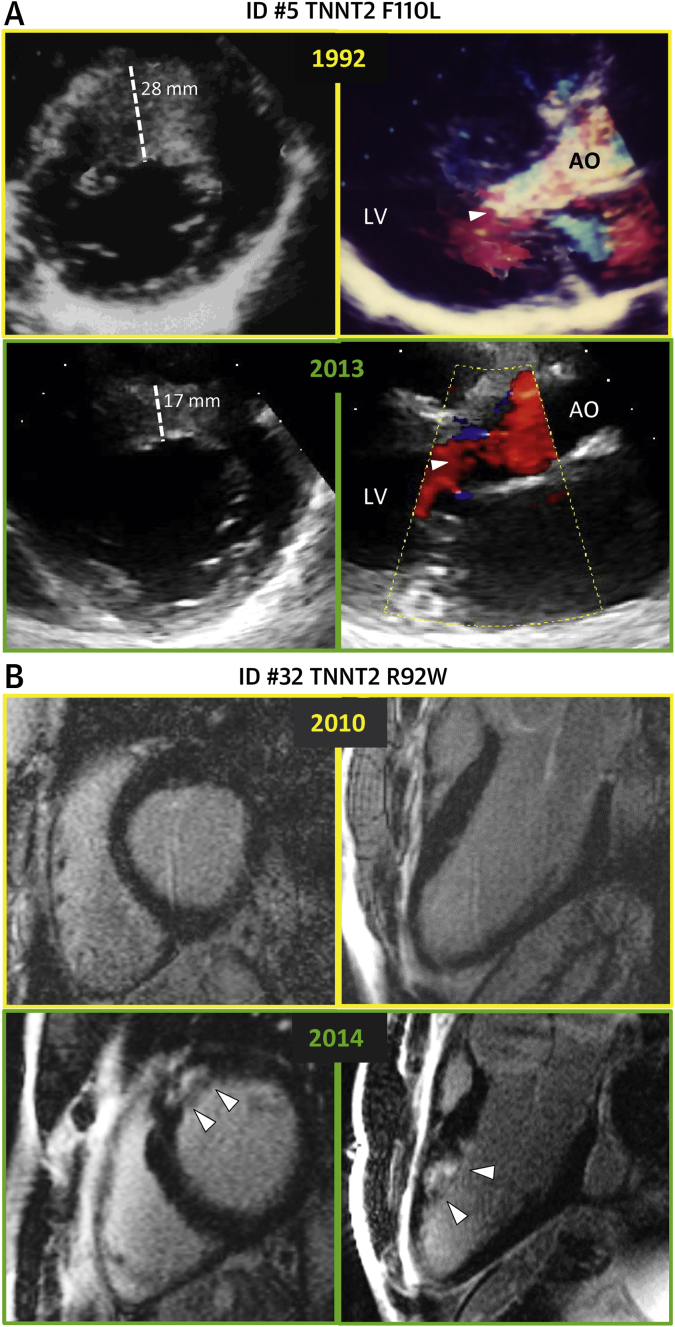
Evidence of Disease Progression in Thin-filament HCM (A) Echocardiographic images from patient ID #5 carrying the TNNT2-F110L mutation. Top: Echocardiographic evaluation at age 16 years. (Left) Parasternal short-axis view showing severe and diffuse anteroseptal LV hypertrophy. (Right) Parasternal long-axis view showing turbulent flow in the LV outflow tract (arrow), caused by severe dynamic obstruction. (Bottom) Similar views from the same patient at age 37 years, showing marked anteroseptal wall thinning and absence of obstruction and increased left atrial size. (B) CMR images from patient ID #32 carrying the TNNT2-R92W mutation. (Top) Cardiac magnetic resonance at age 21 years. Short-axis and 3-chamber views show absence of late gadolinium enhancement (LGE) in the LV wall. (Bottom) Similar views from the same patient at age 25 years. LGE shows extensive fibrous substitution within the anterior septum (arrow), occupying 25% of total LV mass. Abbreviations as in Figure 5.
